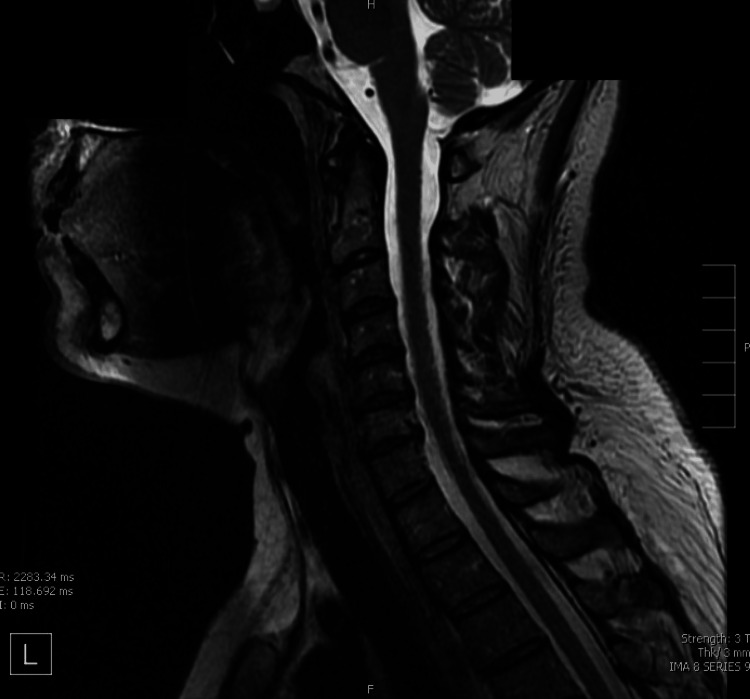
Normal MRI of the Cervical Spine MRI: Magnetic Resonance Imaging
